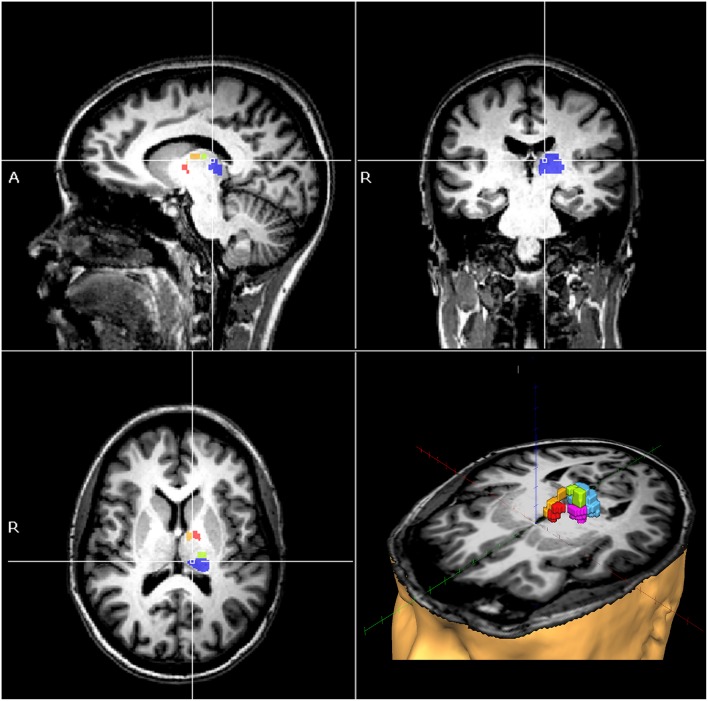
MRI of a single patient as an example, showing the pulvinar (blue), anterior (yellow), ventral anterior (red), dorsal (green), and ventral posterior lateral thalamic nuclei (magenta), where fractional anisotropy values were shown to be independent predictors of verticality perception in post-stroke patients.
